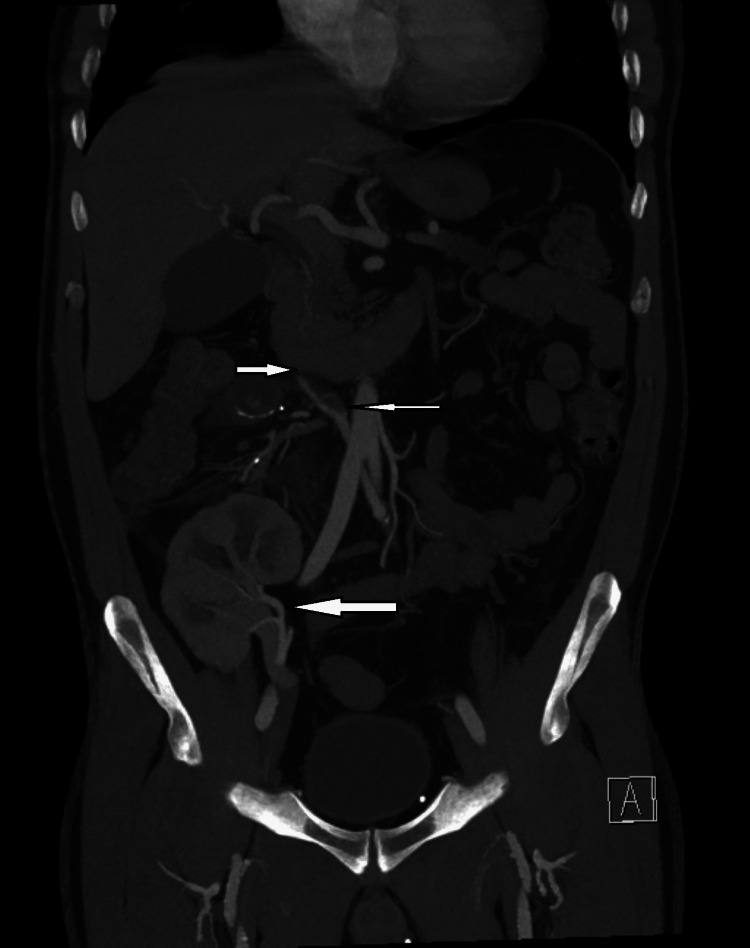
Coronal section of abdominal CT scan showing a thrombosed vascular graft (upper and middle small white arrows) and patent renal graft (lower large single white arrow) CT: computed tomography
